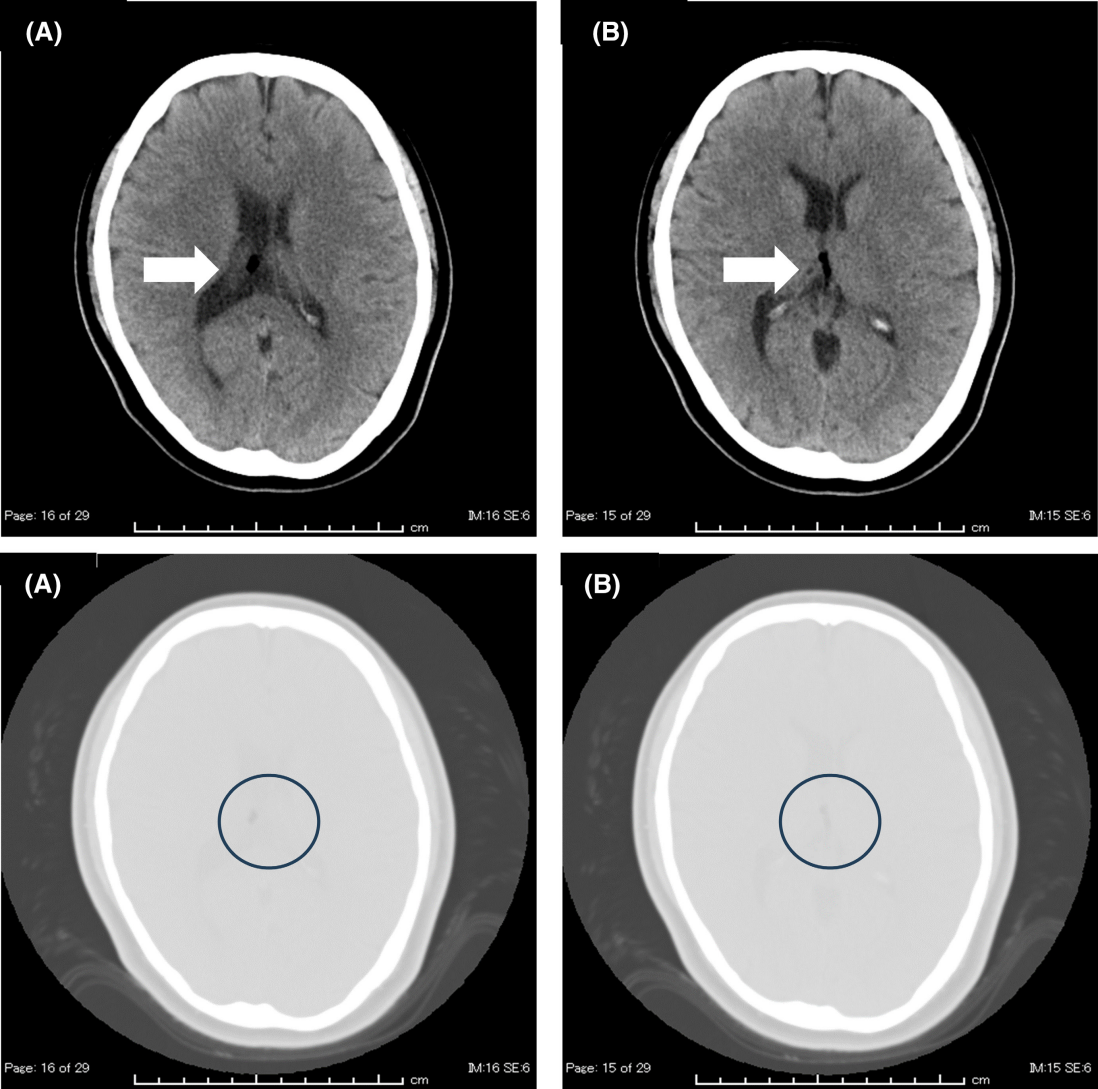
(A, B) Head CT. Upper row: (A) is a lipoma in the right lateral ventricle and (B) in the third ventricle. (white arrow). Lower row: It can be confirmed that both A and B are not air under lung field conditions. (black circle).
